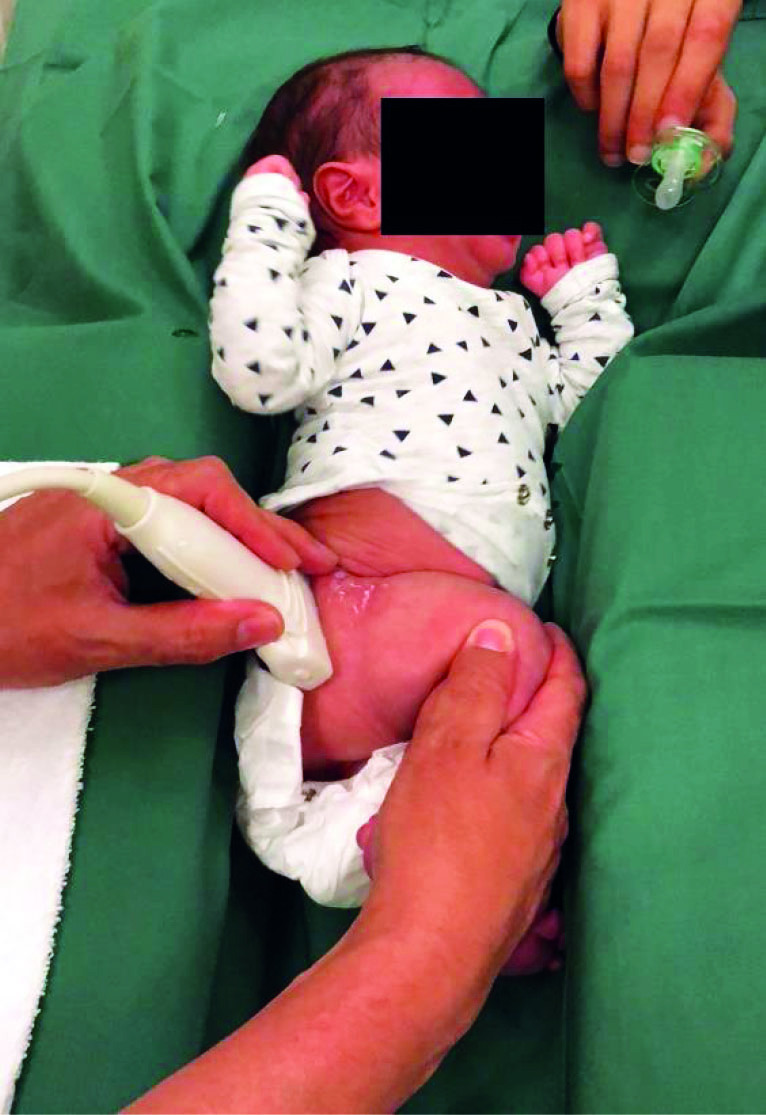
The ultrasound examination with the child in lateral position.
